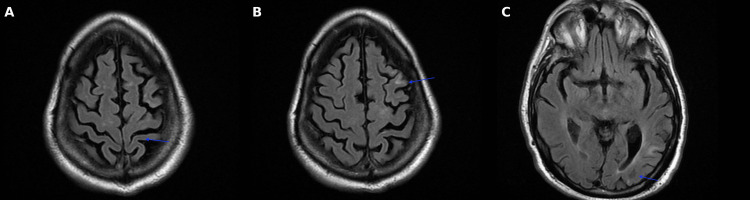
Case 1: (A-C) Corresponding axial MRI brain FLAIR sequence showing the corresponding areas of restricted diffusion seen in the previous figure. FLAIR: fluid attenuated inversion recovery
